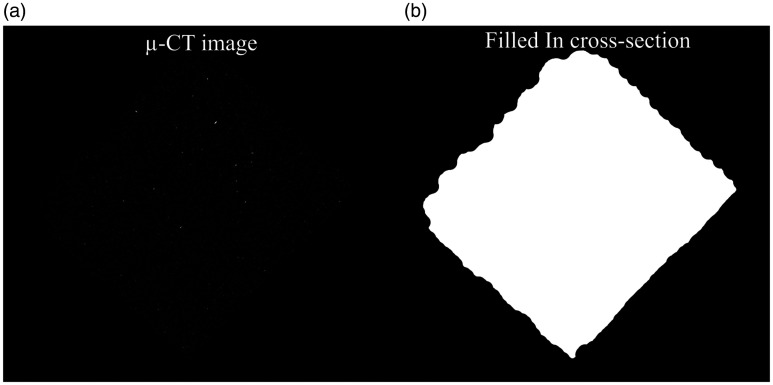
Segmentation of copper PLA sample cross-section: (a) µ-CT image, and (b) filled sample cross-section.
